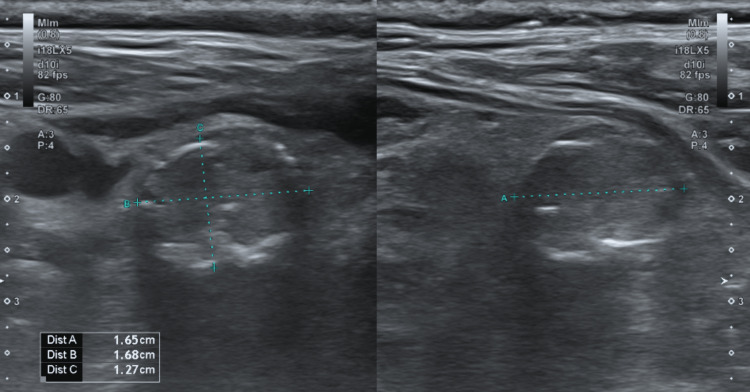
Thyroid nodules seen on an ultrasound of the thyroid.
